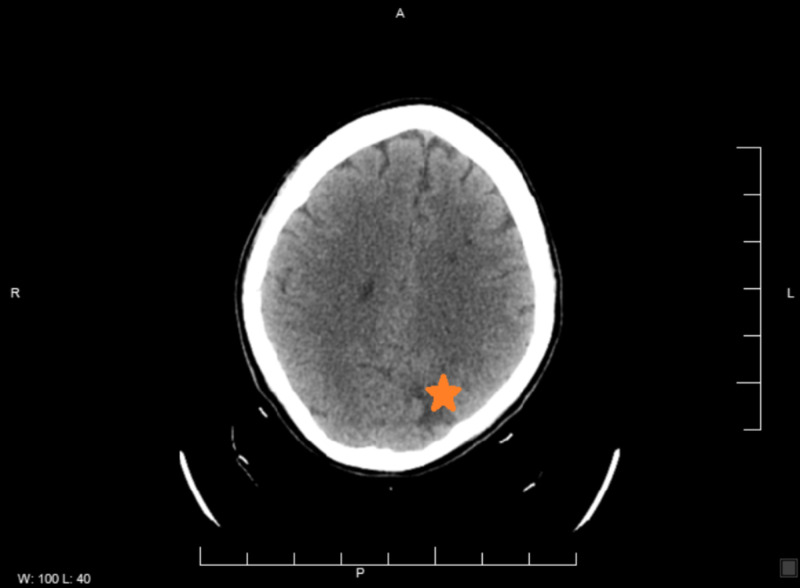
CT brain: low attenuation of the left parietal lobe (orange star)
